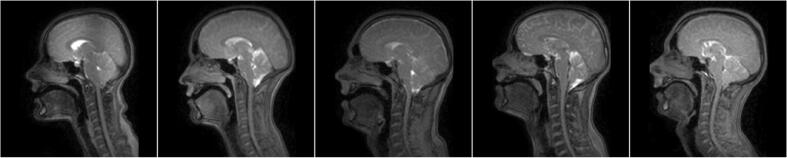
The reference image in each of the five series of dynamic two-dimensional magnetic resonance images. During framework evaluation, these images were used as the moving image for registration purposes.
